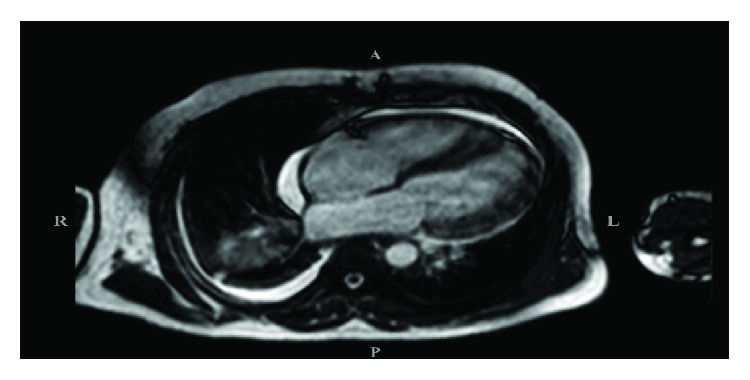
Contrast-enhanced cardiac MRI: contrast late enhancement study shows diffuse patchy contrast late enhancement mostly involving mid- and epicardial layer of myocardium, more at the midlateral wall and inferior wall which can be consistent with myocarditis. Small pericardial effusion.
